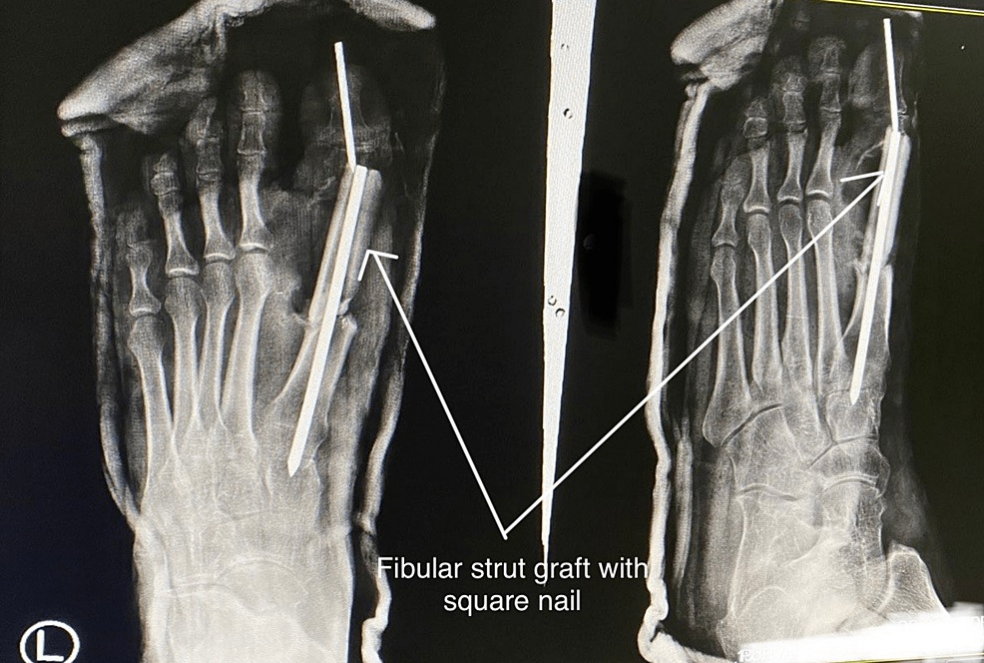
Immediate postoperative X-ray showing a fibular strut graft filling the bony defect fixed with a square nail and Kirschner wire
